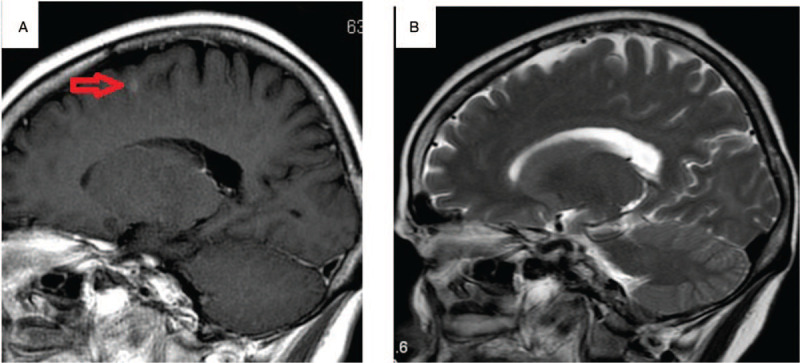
A, Brain metastases in brain MRI. B, Brain metastases have disappeared after ceritinib. MRI = magnetic resonance imaging.
